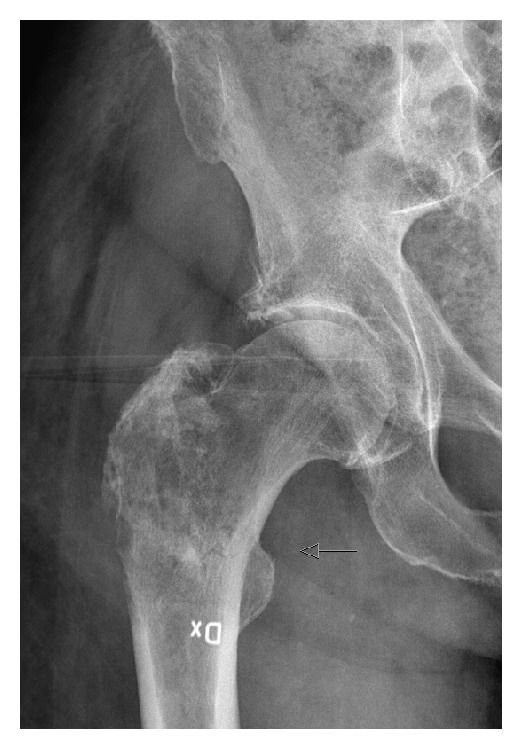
Plain X-ray of the right femur in a patient with advanced metastatic penile cancer reveals a lytic metastasis in the subtrochanteric region and a nondisplaced fracture (arrow).
